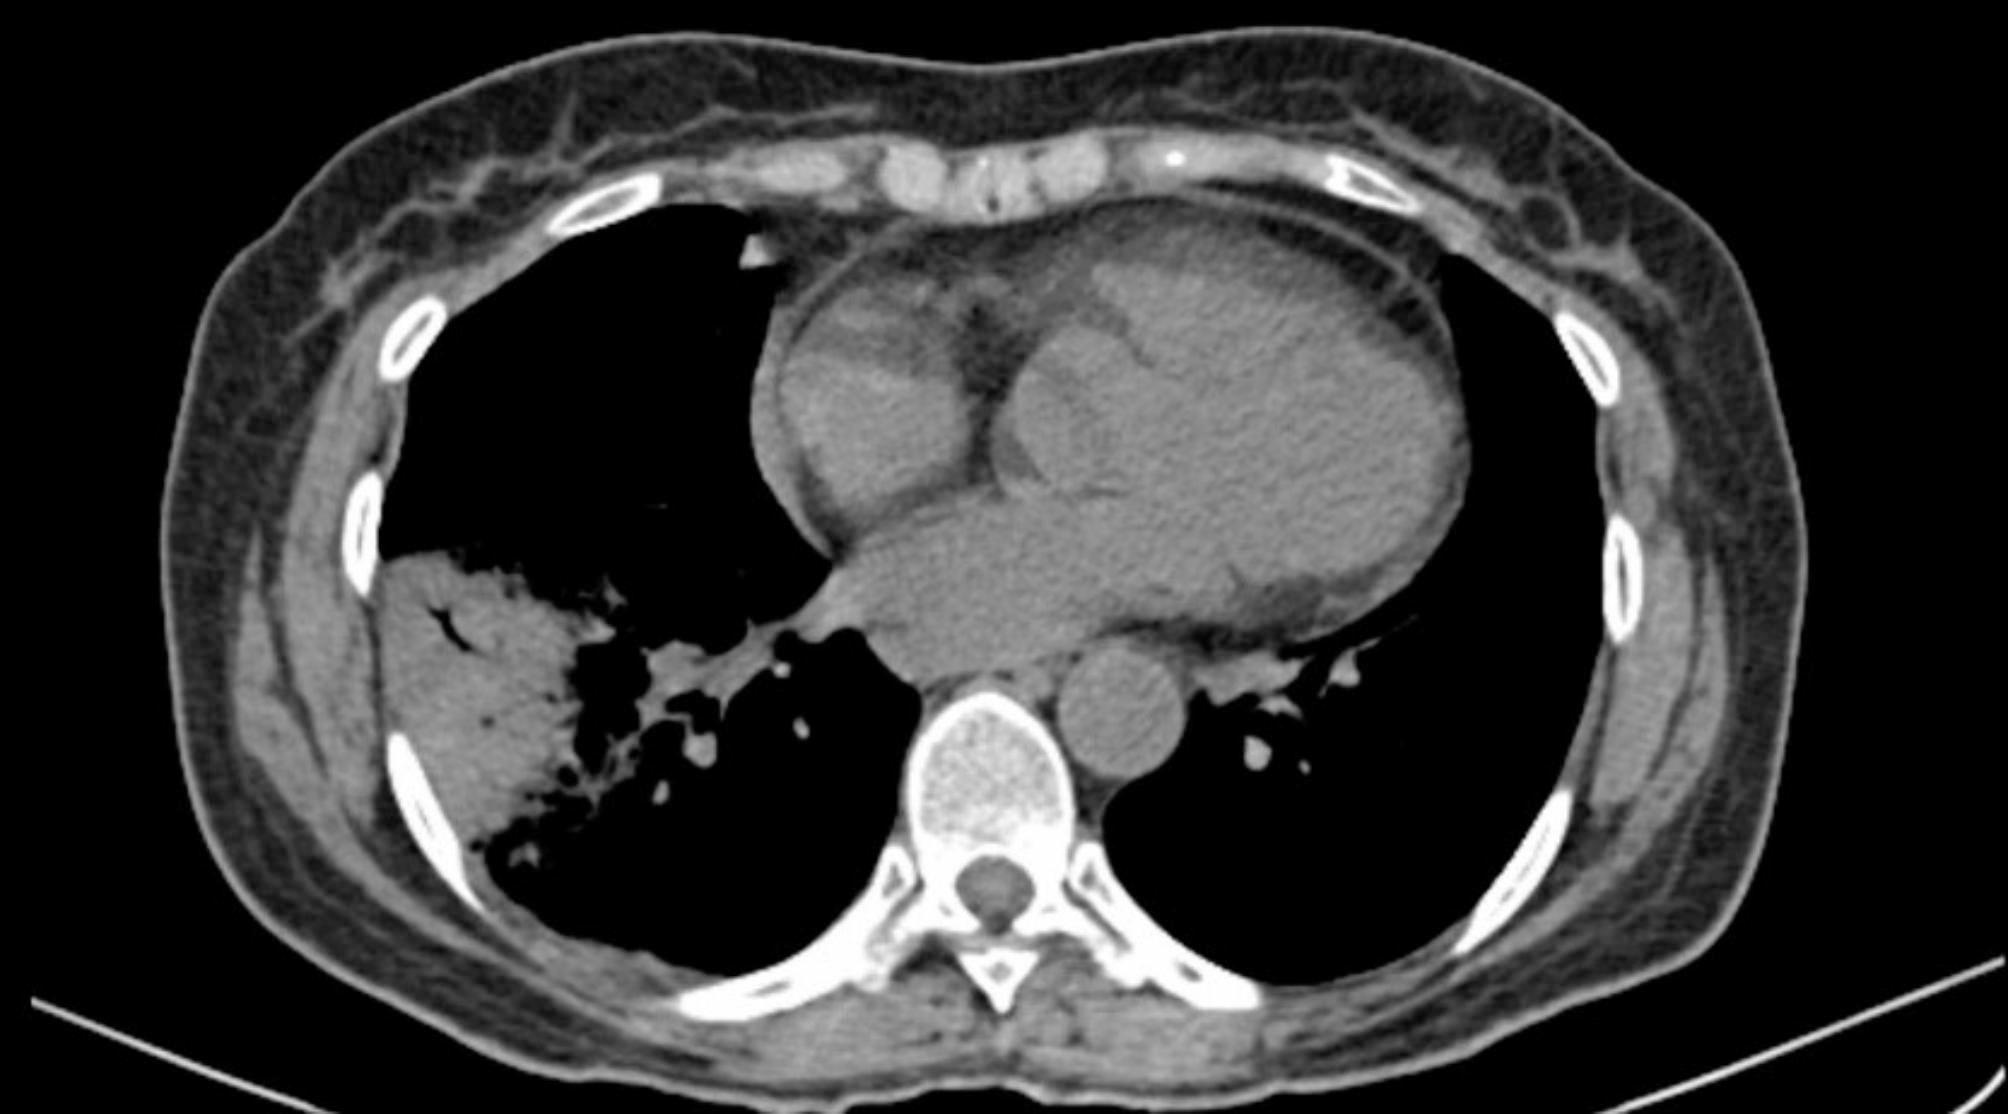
Mediastinal view of lung CT scan shows consolidation and opacity of the Grand Glass of the upper segment and posterior basal of the Right lower lobe
